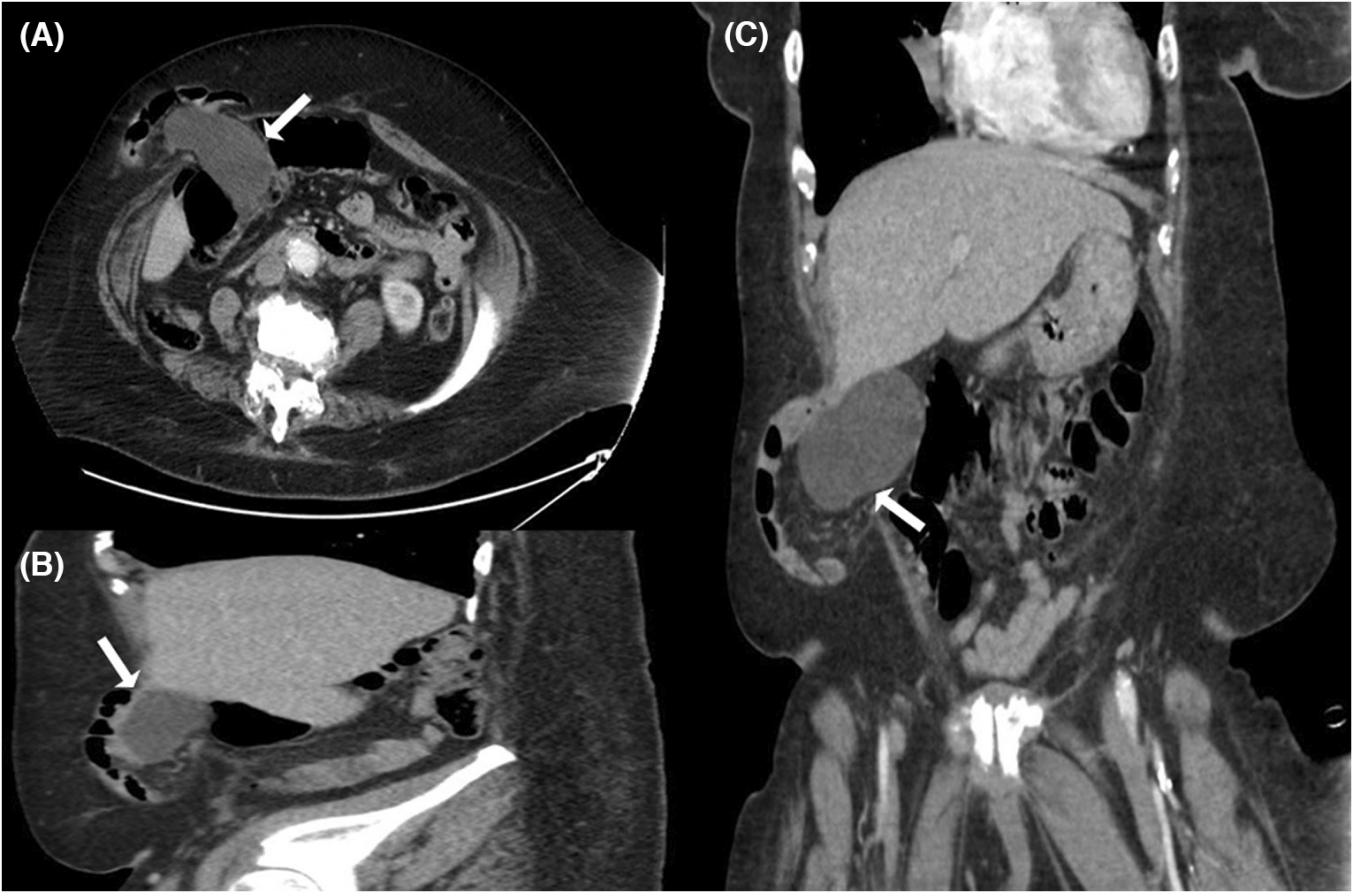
(Panel A–C): Axial, sagittal, and coronal computed tomography (CT) images of the abdomen and pelvis showing a right lower quadrant ventral hernia containing non‐obstructed, non‐distended small intestinal loops and the dome of a distended gallbladder.
